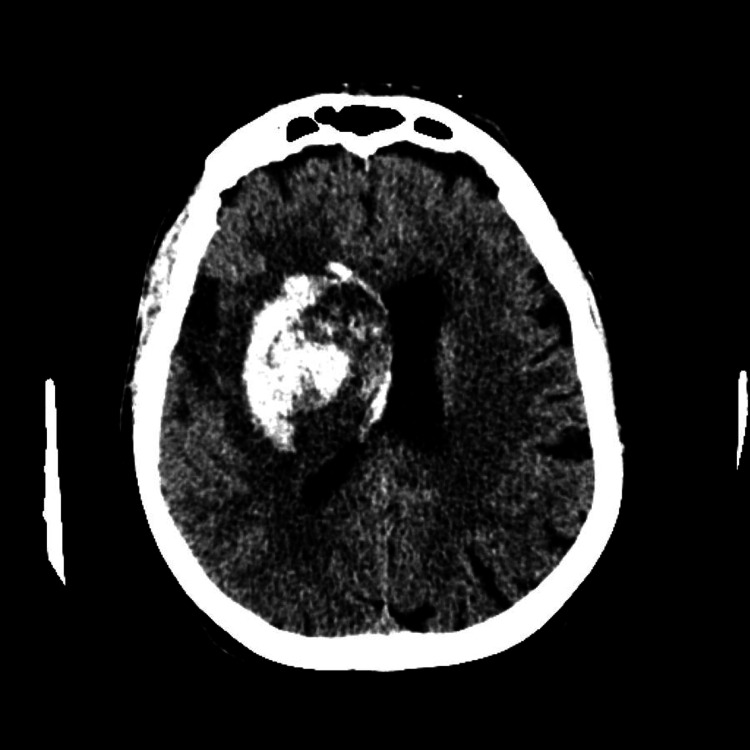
Head CT of right basal ganglia and temporal lobe intraparenchymal hemorrhage with intraventricular hemorrhage and midline shift CT: Computed Tomography
